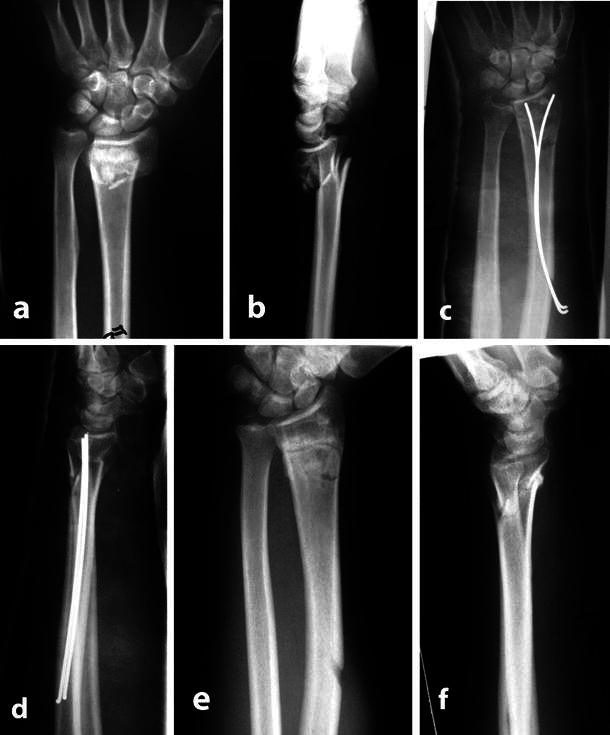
45-year-old male with (a and b) Frykman’s type II (AO: A 3-2) fracture of distal-end left radius; (c and d) post-operative radiographs showing the blunt ends of K-wires supporting the subchondral bone; (e and f) radiographs after removal of K-wires
